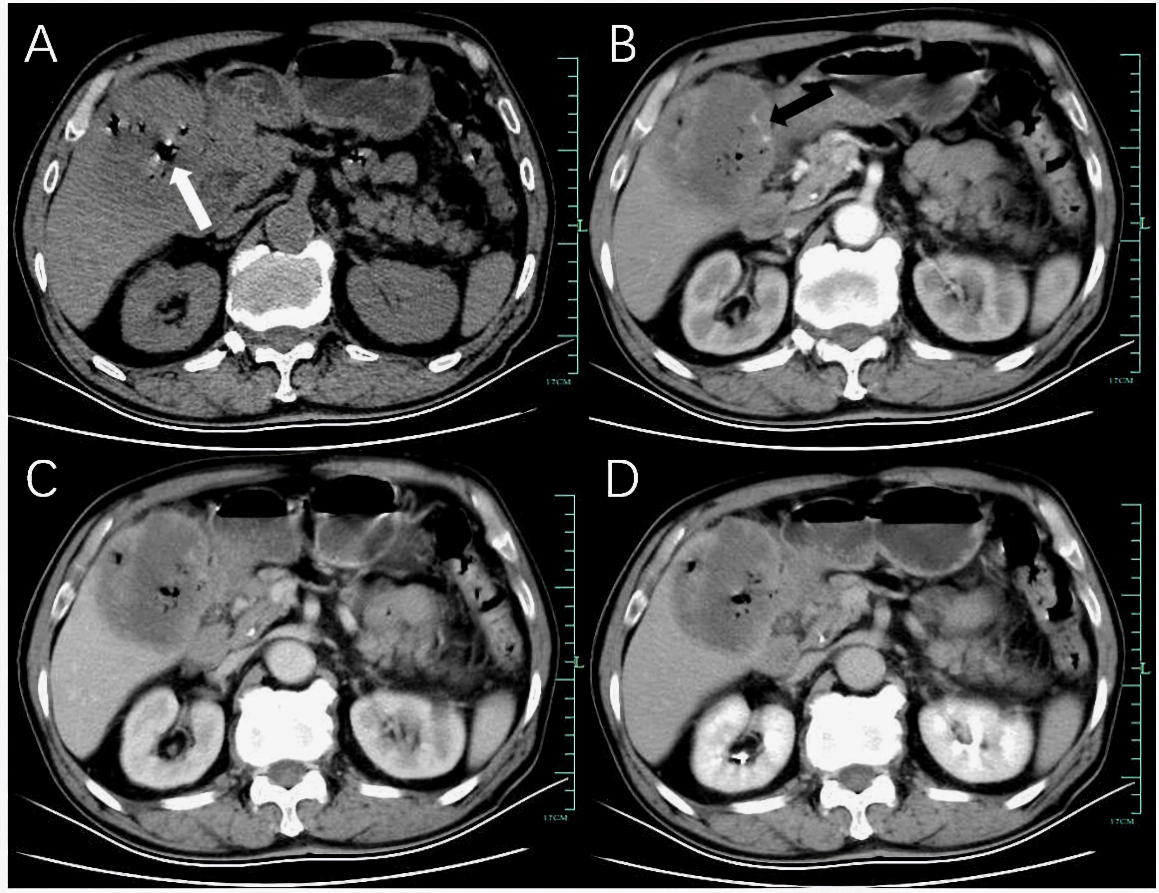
(A) Computed Tomography (CT) demonstrated a low-density mass in the right lobe of the liver, with post-puncture gas accumulation (white arrow). (B) In the arterial phase, the mass margins showed uneven enhancement, with small blood vessels originating from the hepatic artery noted (black arrow). (C and D) In the portal venous and delayed phases, the mass margins remained enhanced.
